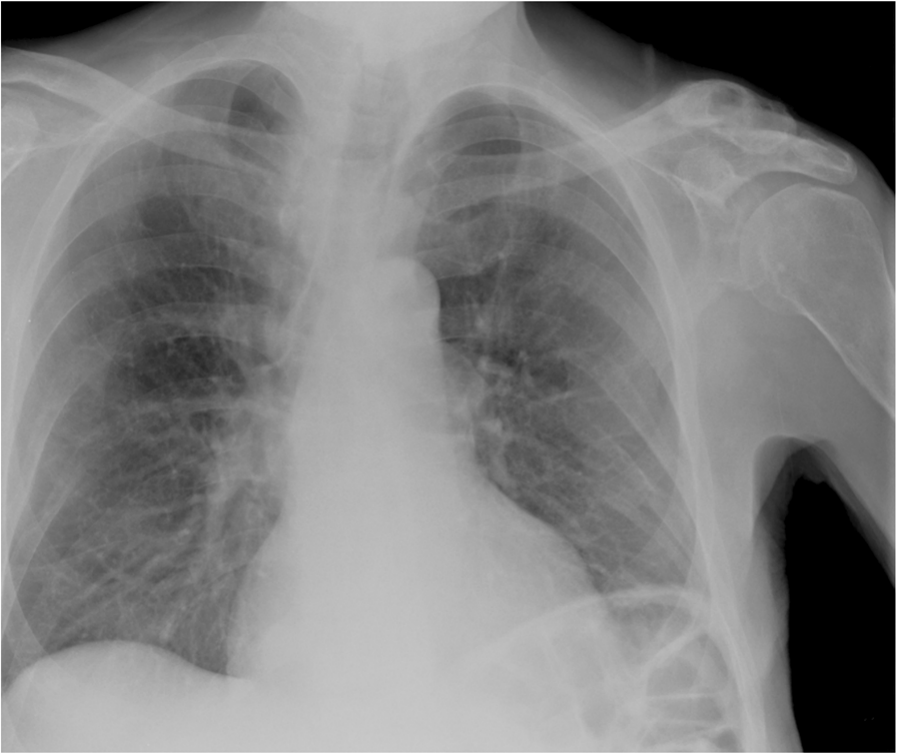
Chest radiograph of the same patient showed an expansile mixed lytic/sclerotic lesion in the lateral aspect of the left clavicle
